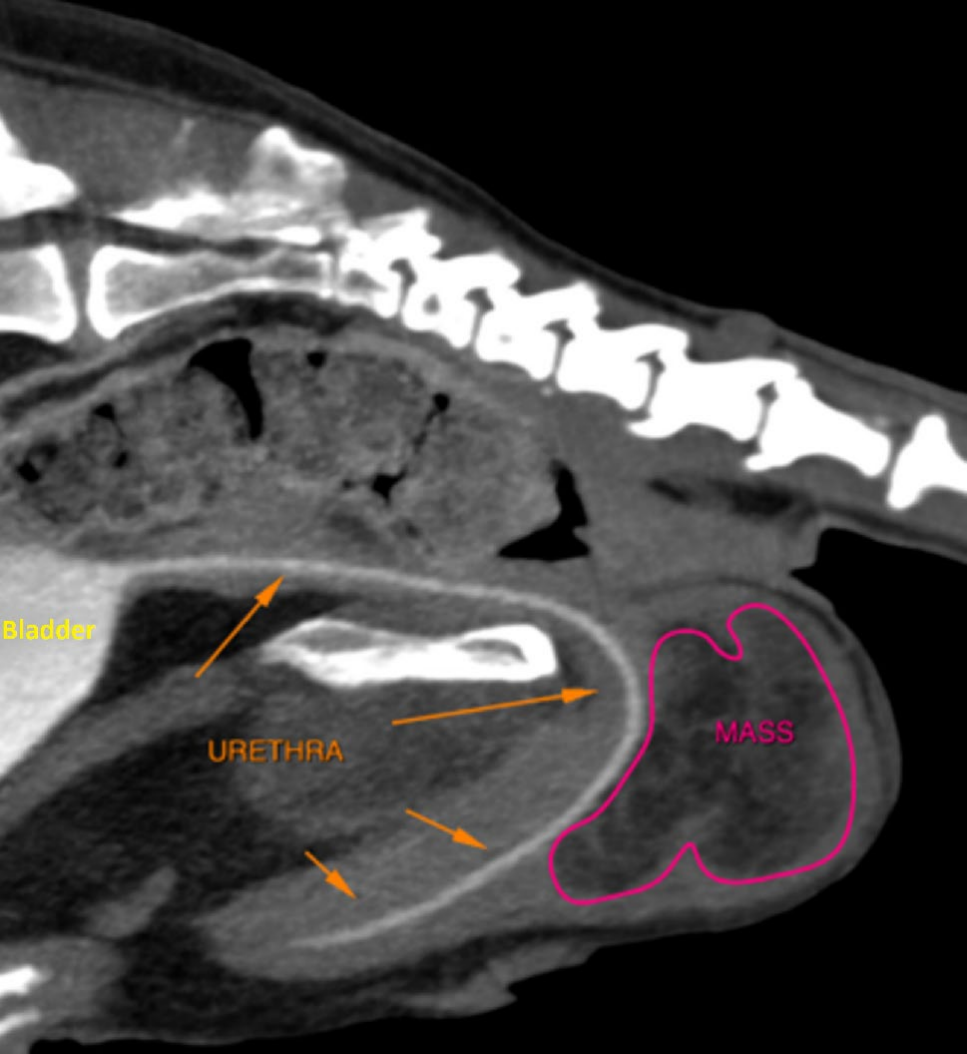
The extent of the tumour on computed tomography. Sagittal view of the pelvic region showing an inhomogeneous mass with heterogeneous fat attenuation and soft tissue streaks caudal to the perineal urethra
